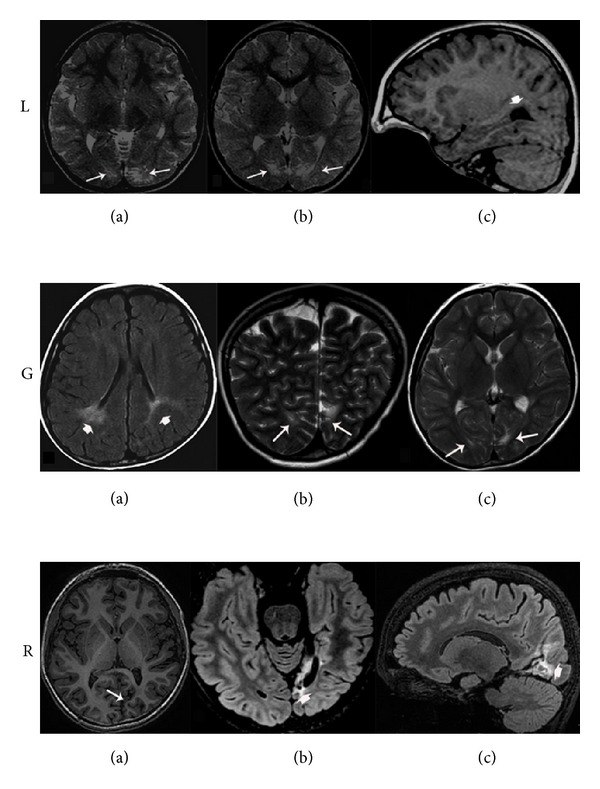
MRI scan for each patient. L.: (a), (b) Brain MRI (axial T2 and sagittal T1 weighted images) showing bilateral periventricular hyperintensities in the occipital lobes (white arrows) and dilatation of the occipital horn of the lateral ventricle (white arrowhead). G.: (a)–(c). Brain MRI (axial FLAIR, coronal and axial T2 weighted images) showing bilateral periventricular hyperintensities in the parietooccipital areas (see white arrowheads and arrows). R.: (a) Brain MRI (axial T1 weighted image) showing cortical-subcortical atrophy and abnormal cortical sulcation in the left temporooccipital area (white arrow). (b), (c). Brain MRI (axial and sagittal FLAIR weighted images) showing increased signal intensity in the left calcarine cortex and dilatation of the occipital horn of the left lateral ventricle (white arrowheads).
